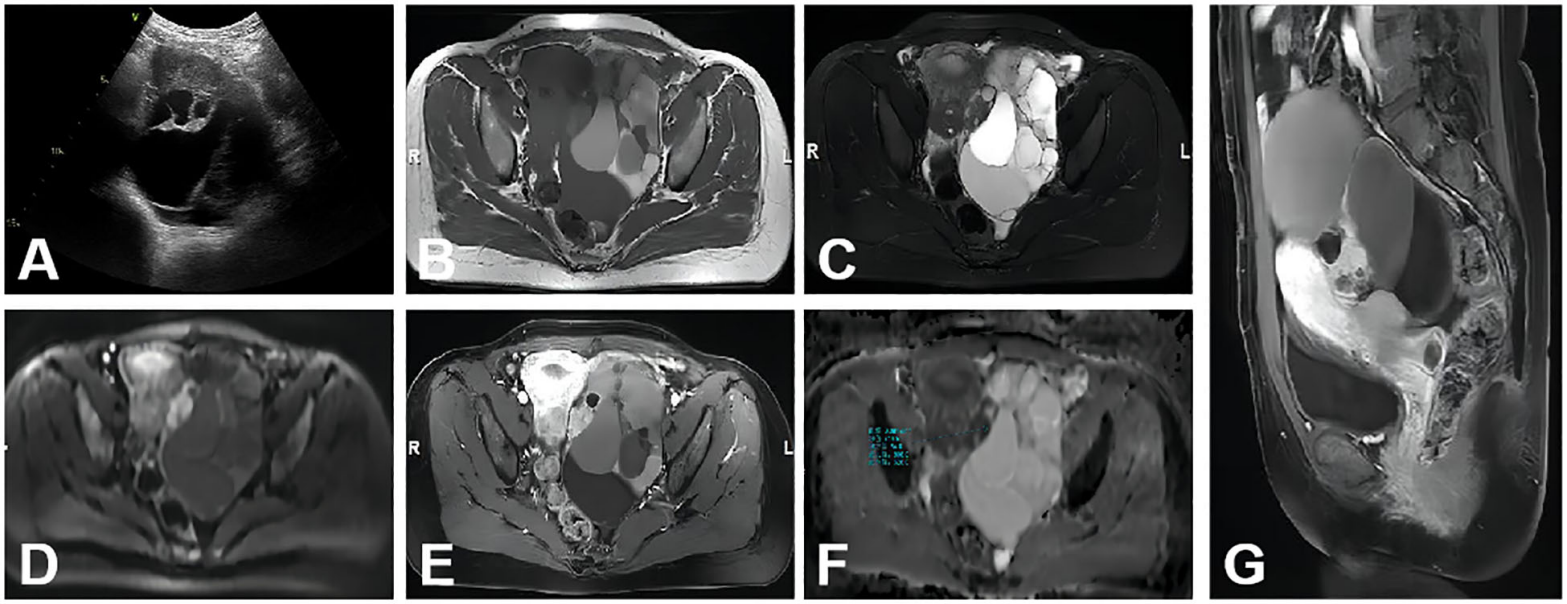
A 43-year-old woman with stage I MC on the left ovary. (A) A multilocular and predominantly cystic mass with thickened septa was detected by ultrasound examination. (B–D) A lobulated multi-cystic mass with mural nodule and irregular septations; the mural nodules showed mainly isointense, hyperintense, and hyperintense signal intensities on axial T1WI, T2WI, and DWI (b=1,000 s/mm^2^), respectively. (E) Axial contrast-enhanced T1WI showed that the mural nodule and the irregular septation were of moderate enhancement. (F) On the ADC map, the mean ADC value was 0.719 × 10^−3^ mm^2^/s. (G) Sagittal contrast-enhanced T1WI showed the mass was similar to (E).
